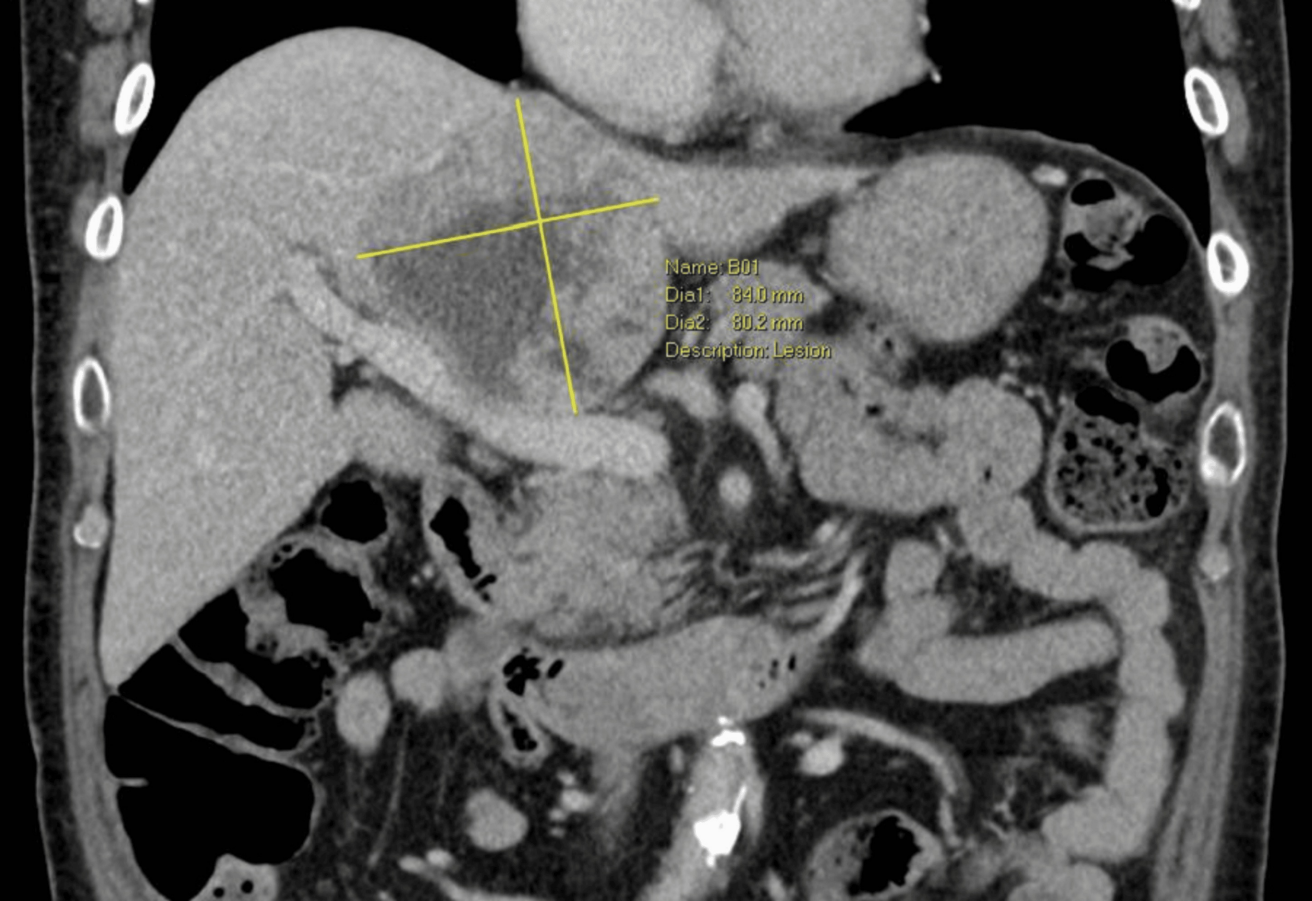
Coronal computed tomography (CT) venous phase demonstrating a large, centrally located tumour close to the portal vein.
